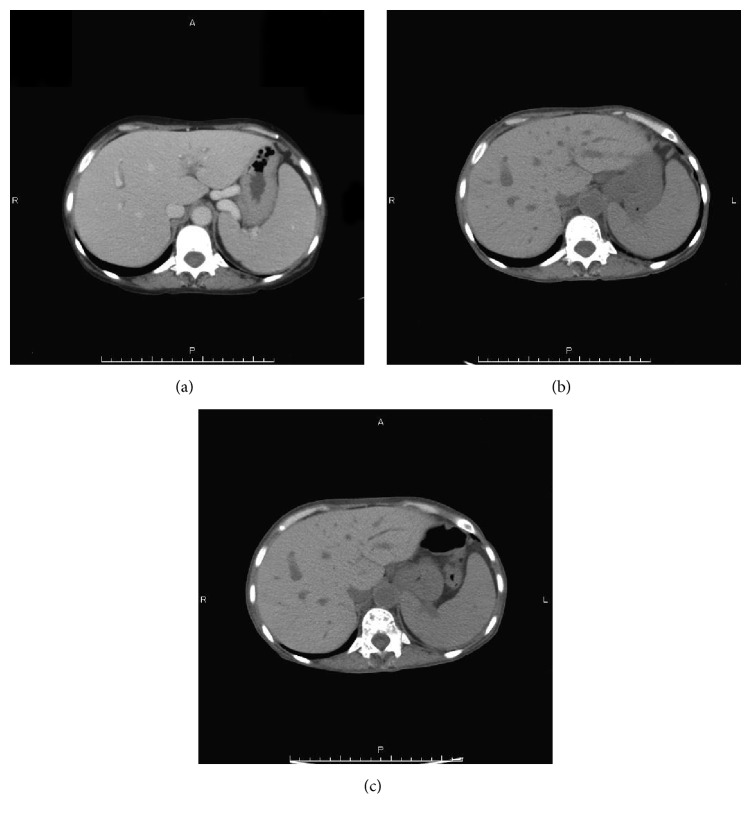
Case  2.* CT. (a) Before HCT, the CT showed severe splenomegaly. (b, c) After HCT, the massive splenomegaly was reduced. (b) Three months. (c) Six months.
